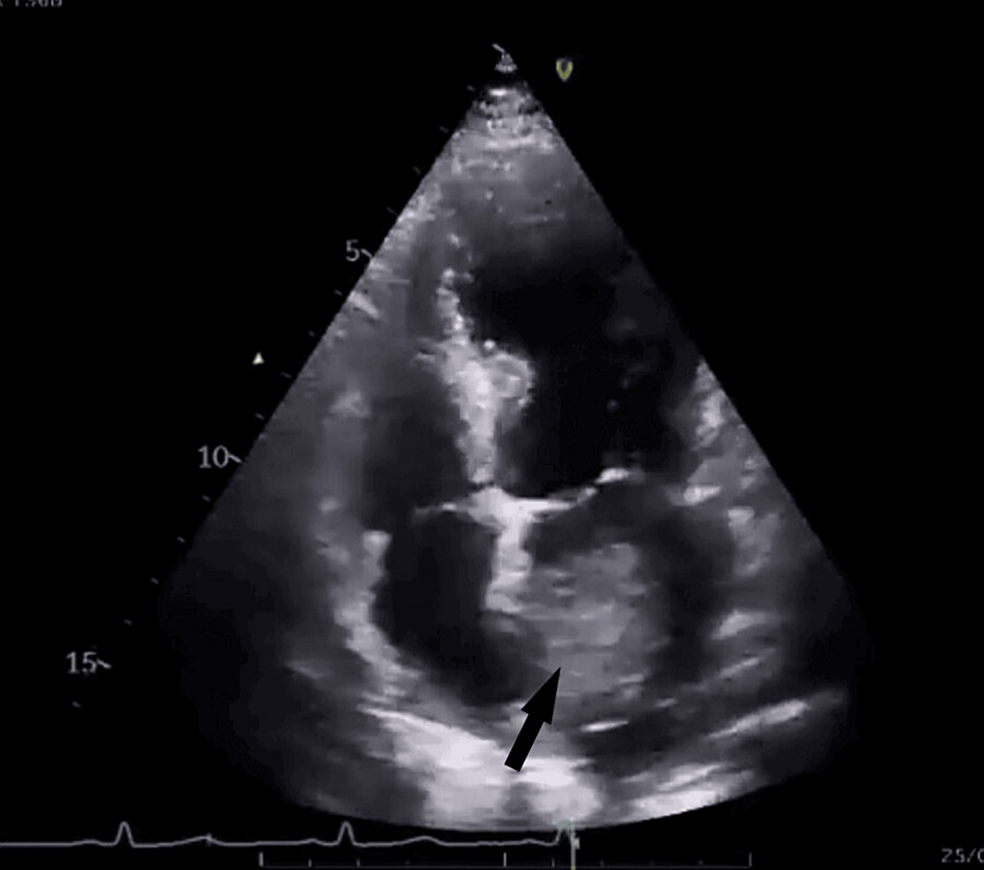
Echocardiogram demonstrates the presence of a left atrial mass, causing left ventricular inflow obstruction.
